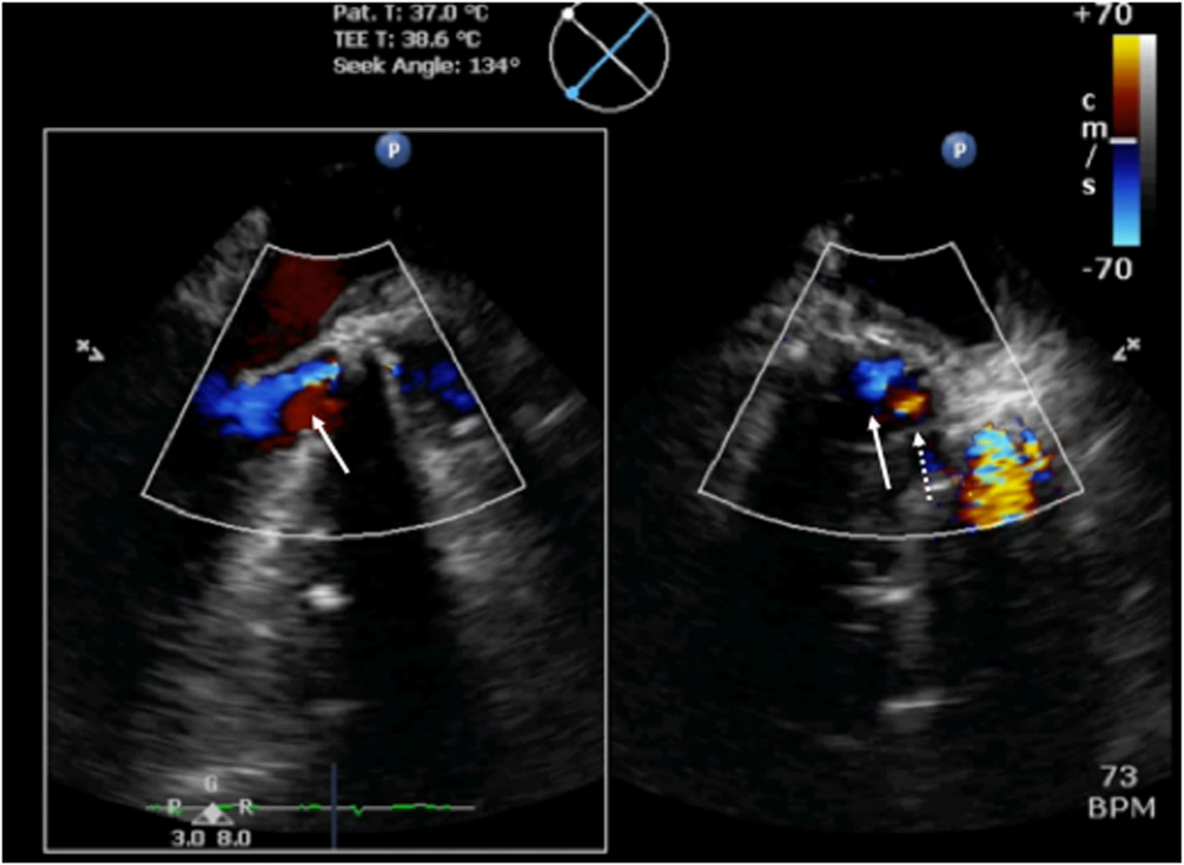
Using LIVE xPlane, a mid-esophageal aortic valve long-axis transesophageal echocardiography image (left image) and aortic valve short-axis transesophageal echocardiography image (right image) were taken, with the image inverted from right to left. Acceleration flow was recognized in the non-coronary cusp of the intra-sewing ring white arrow indicates aortic regurgitation and white dotted arrow indicates its acceleration flow
